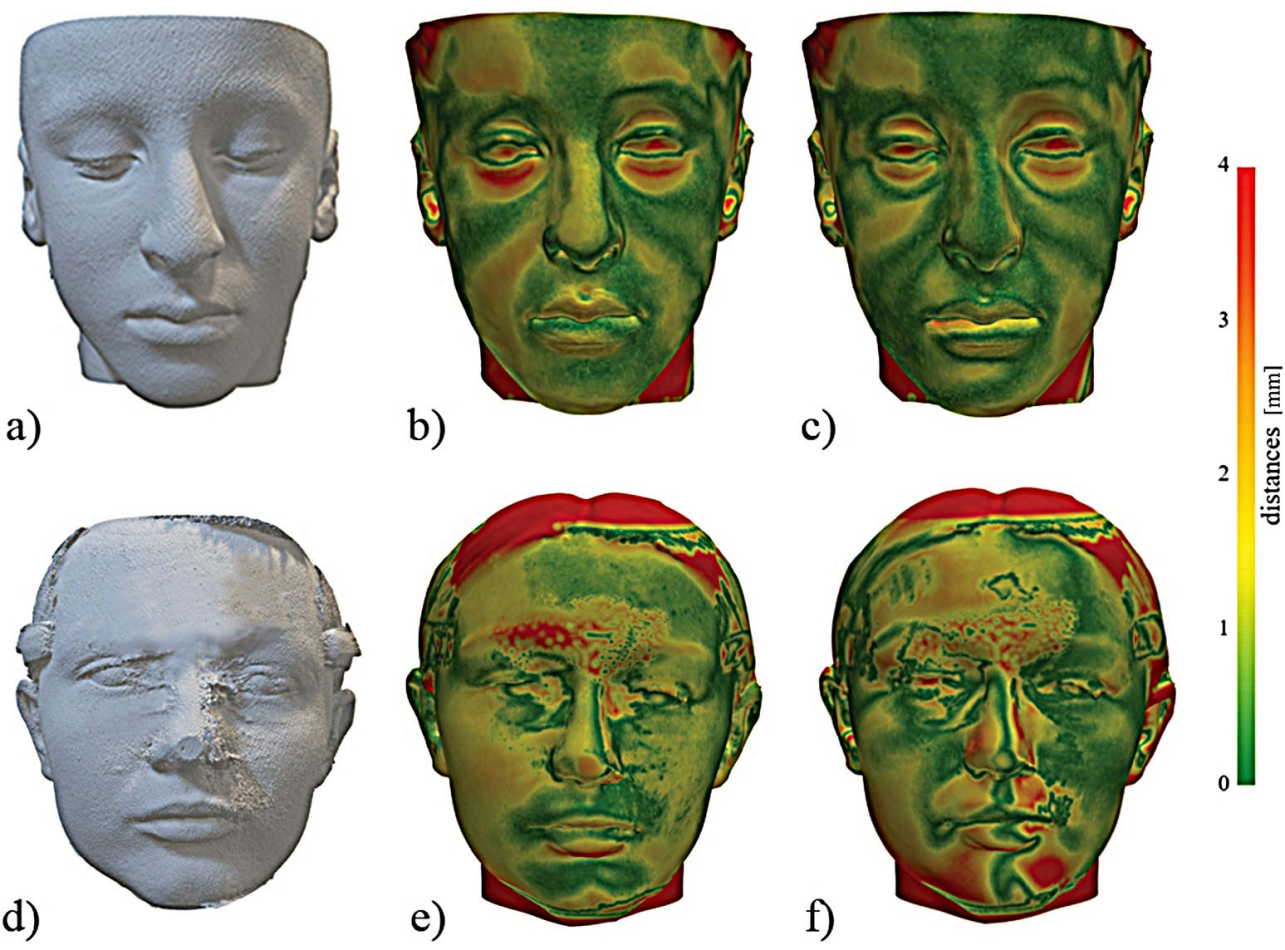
Distance map between surface rendering and registered facial scan a) Multislice computed tomography (MSCT) surface rendering b) Distance map between automatically registered facial scan and MSCT surface rendering c) Distance map between semi-automatically registered facial scan and MSCT surface rendering d) Cone beam computed tomography (CBCT) surface rendering e) Distance map between automatically registered facial scan and CBCT surface rendering f) Distance map between semi-automatically registered facial scan and CBCT surface rendering, A-C is a 14-year old male patient, D-F is a 15-year old female patient
